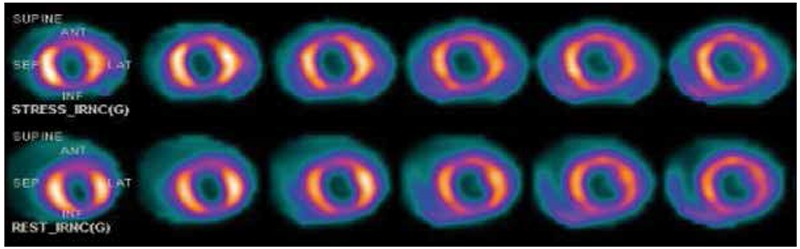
The view of a 50-year-old male patient with myocardial perfusion scintigraphy. Myocardial perfusion scintigraphy in all areas are monitored reduced and heterogeneous uptake. Three-vessel disease was detected in the patient’s coronary angiography
